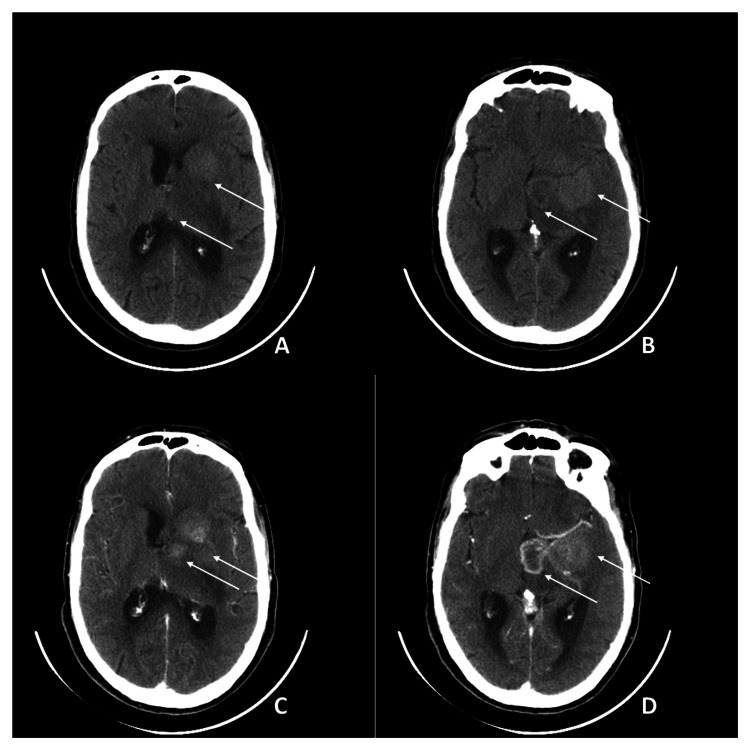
Brain CT scan revealing a new left hemispheric expansile lesion with pronounced edema, hydrocephalus, and midline shift. (A) Axial view highlighting the left medial temporal lesion (arrows), showing hyperdense areas and moderate heterogeneous enhancement. (B) Axial view focusing on the anterior thalamo-capsular and subthalamic lesions (arrows) with irregular ring enhancement and a likely necrotic core. (C) Axial view demonstrating significant edema extending inferiorly to the left mesencephalon and pons (arrows) contributing to mass effect and midline shift. (D) Axial view depicting the left anterior nucleo-capsular lesion (arrows) showing heterogeneous enhancement and a likely necrotic central area in association with hydrocephalus and midline shift CT: computed tomography
